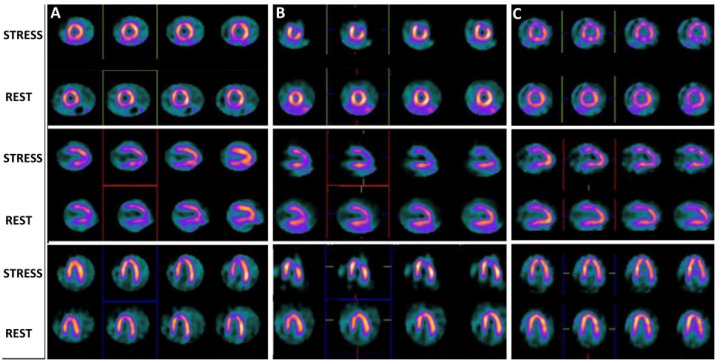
(A): Normal pattern of left ventricle stress and rest myocardial perfusion in a patient affected by Kawasaki disease. (B): Reversible myocardial perfusion defect (as for ischemia) in the antero-lateral wall of the left ventricle in a patient with Kawasaki disease. (C): Stress/rest myocardial perfusion scintigraphy showed mild multiple regional abnormalities of myocardial perfusion in a heart transplant patient.
